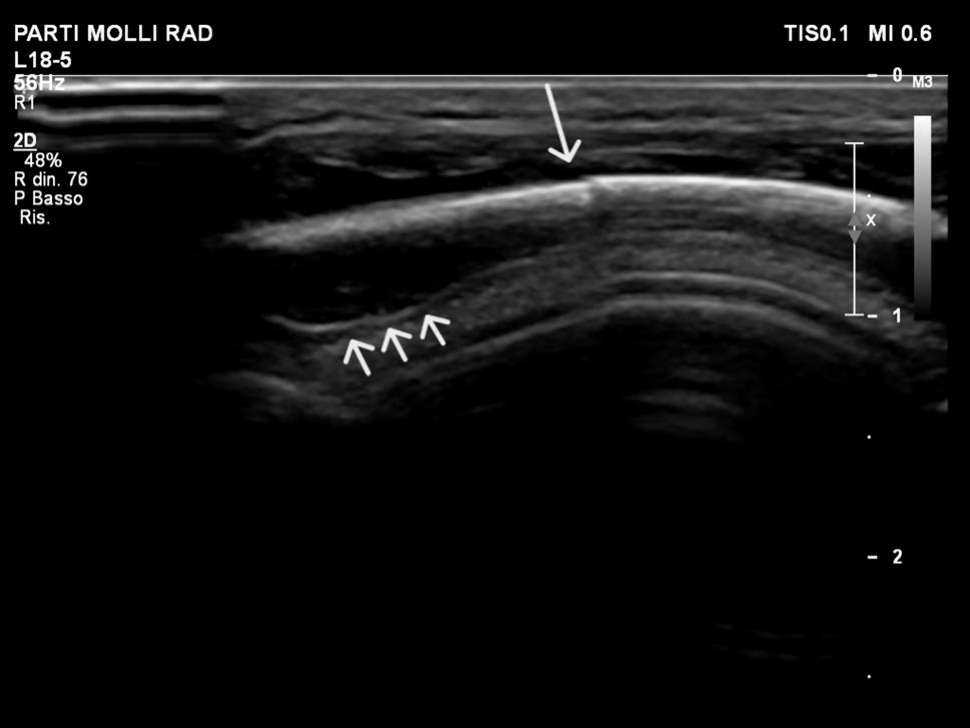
Point-of-care Ultrasound performed using a very-high frequency (18–5 MHz) linear array transducer. The longitudinal view reveals a linear skull fracture (long arrow), accompanied by a small subdural hematoma (short arrows), corresponding to the clinically apparent parietal swelling. The image is obtained in the coronal plane, providing a visualization of the fracture and its spatial relationship with the surrounding tissues
